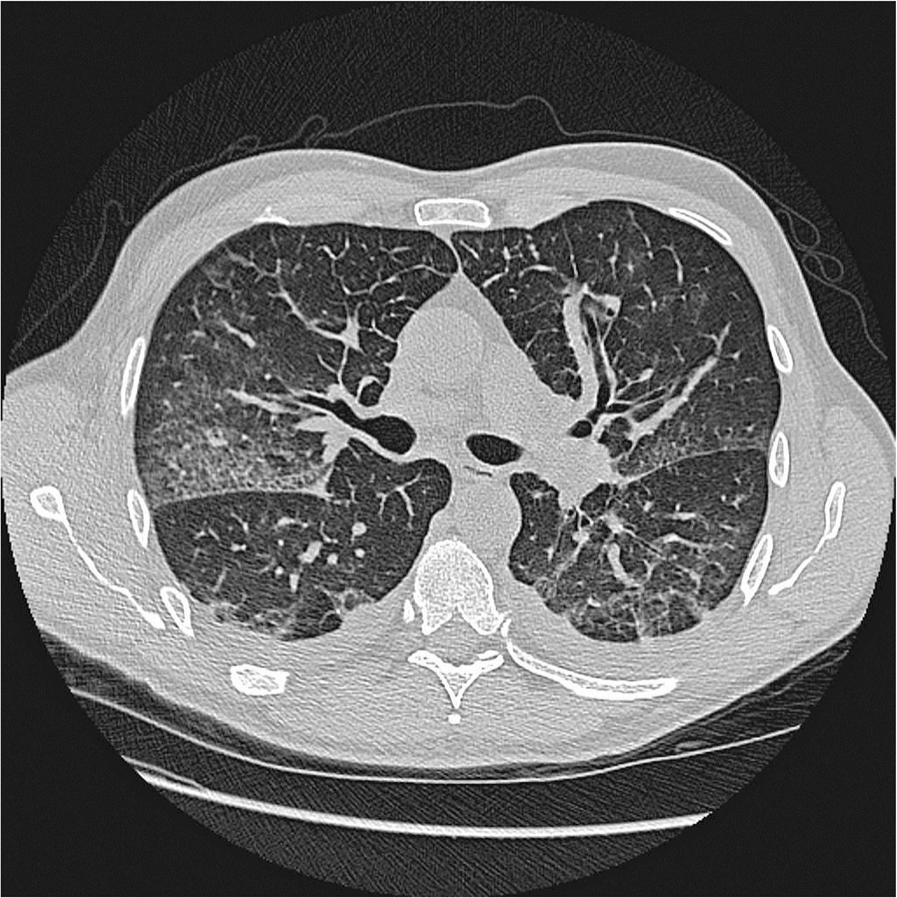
Chest high-resolution computed tomography image from a patient with Puumala hantavirus infection. Investigation was performed four days post onset of disease and shows bilateral pleural effusion and pulmonary oedema in a previously healthy non-smoking patient with pronounced clinical lung manifestations.
